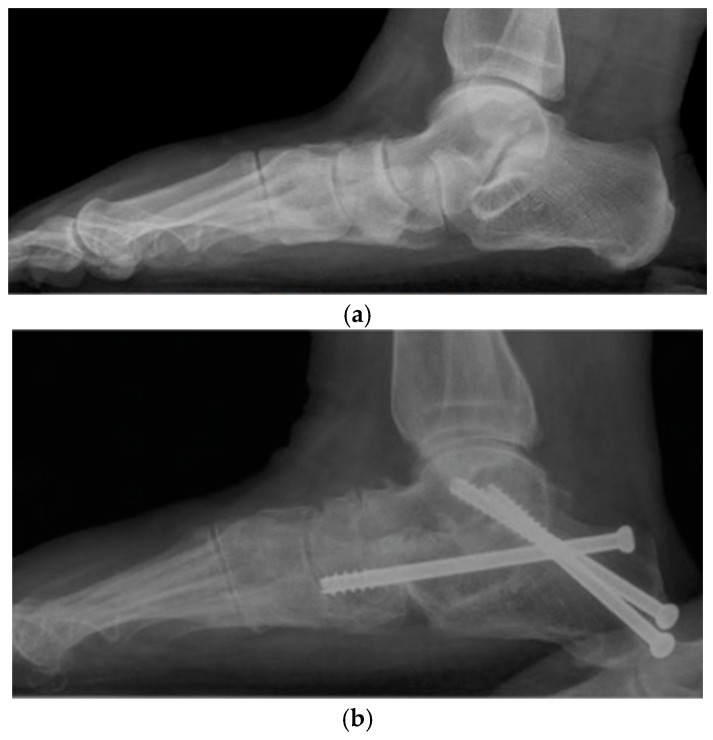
(a) Standing lateral radiograph of the right foot of a 48-year-old patient whose flatfoot recently became painful following a marked increase in body weight. (b) A triple arthrodesis was performed, with partial radiographic correction of the flatfoot and disappearance of pain.
